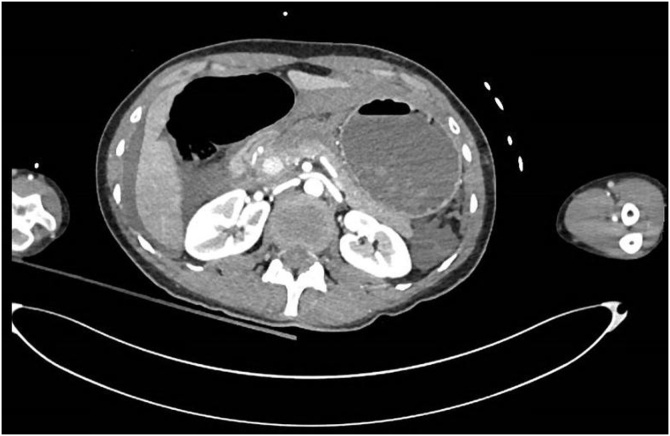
Axial CT demonstrating a distended stomach and free intra-abdominal fluid.
